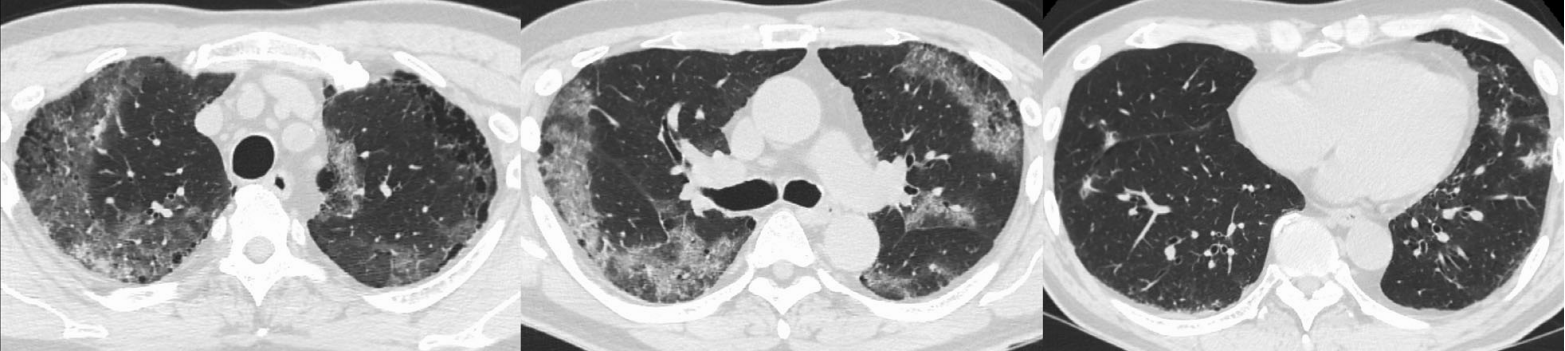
Chest computed tomography (CT) in case 2 showed peripheral consolidation predominant in the upper lobes.
